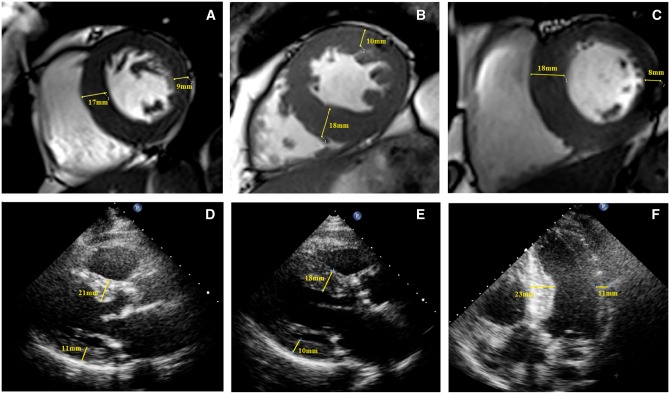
Asymmetrical wall thickening on both magnetic resonance and echocardiography. Images demonstrating asymmetric wall thickening in patients with aortic stenosis. Cardiovascular magnetic resonance short-axis cine images showing an abnormally thickened septum: in a patient with asymmetric remodelling (*A*) and two subjects with asymmetric hypertrophy (*B*) and (*C*). Echocardiographic parasternal long-axis images demonstrating thickening of the septum in two further patients with asymmetric remodelling (*D*) and (*E*). Echocardiographic apical 4-chamber image in a subject with asymmetric hypertrophy (*F*).
